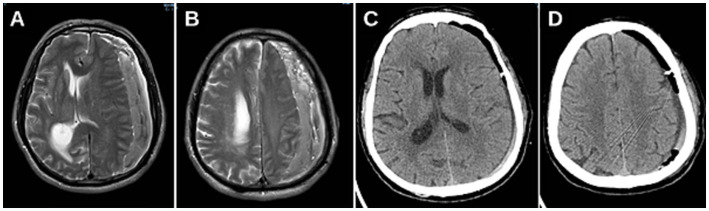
Radiological presentation and surgical outcome for case 2. (A,B) Preoperative imaging revealing a large, complex crescentic collection characterized by significant internal layering, separation, and mass effect with midline shift. (C,D) Postoperative CT scan shows 95% hematoma volume reduction, resolution of the compartmentalization, and restoration of normal intracranial anatomy following the triple-modality protocol.
